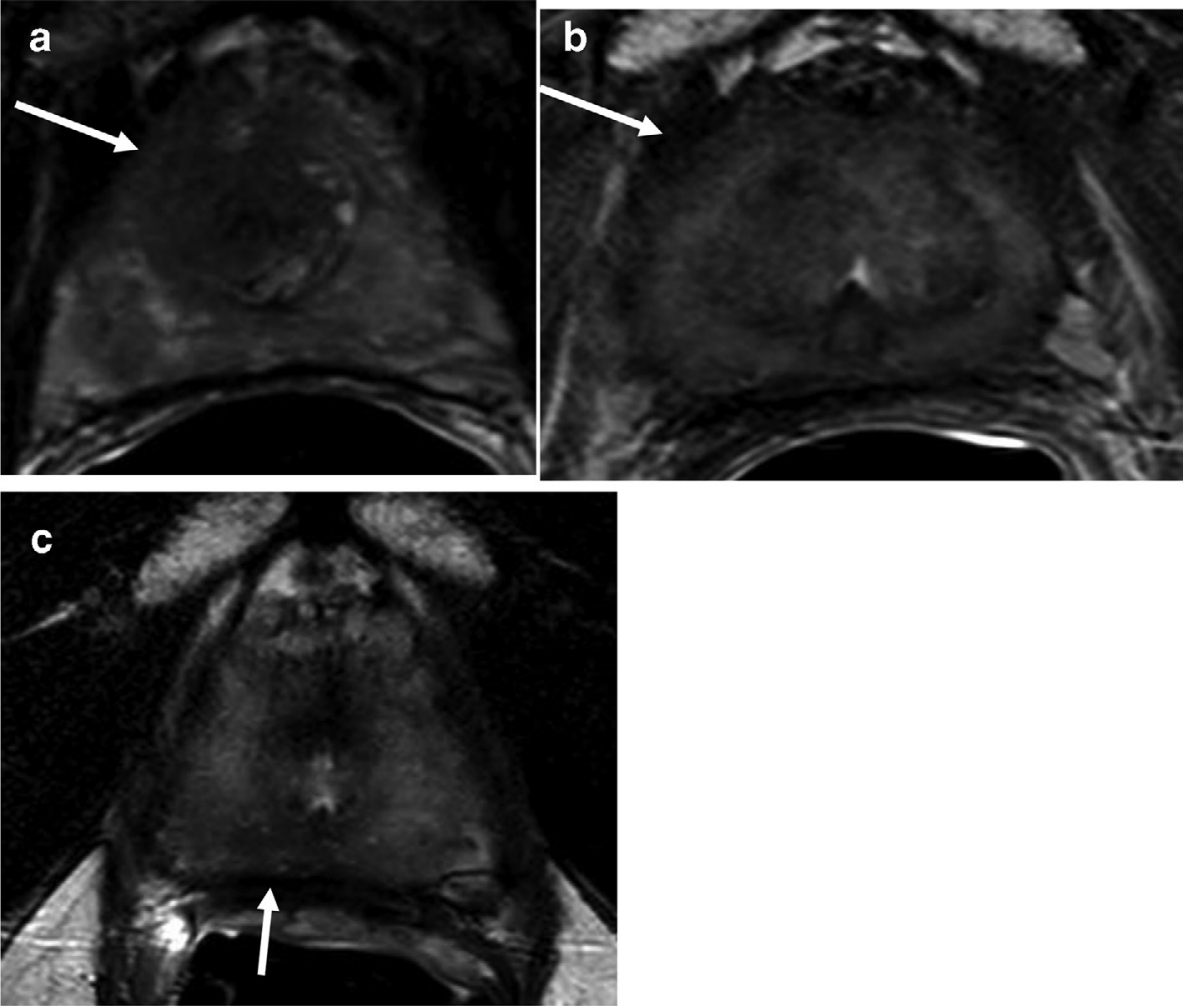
Axial T2W MRI shows an ill defined lesion affecting the right apical-mid anterior peripheral and transition zones prior to treatment (A). Post-treatment axial T2W MRI shows diffuse iso-hypointense signal pattern secondary to treatment effects, with findings consistent with treatment response and low suspicion of residual disease (B). Axial T2W MRI after biochemical recurrence shows diffuse iso-hypointense signal pattern secondary to treatment effects along with a hypointense lesion in the midline to right apical peripheral zone, suspicious for recurrent prostate cancer (C).
